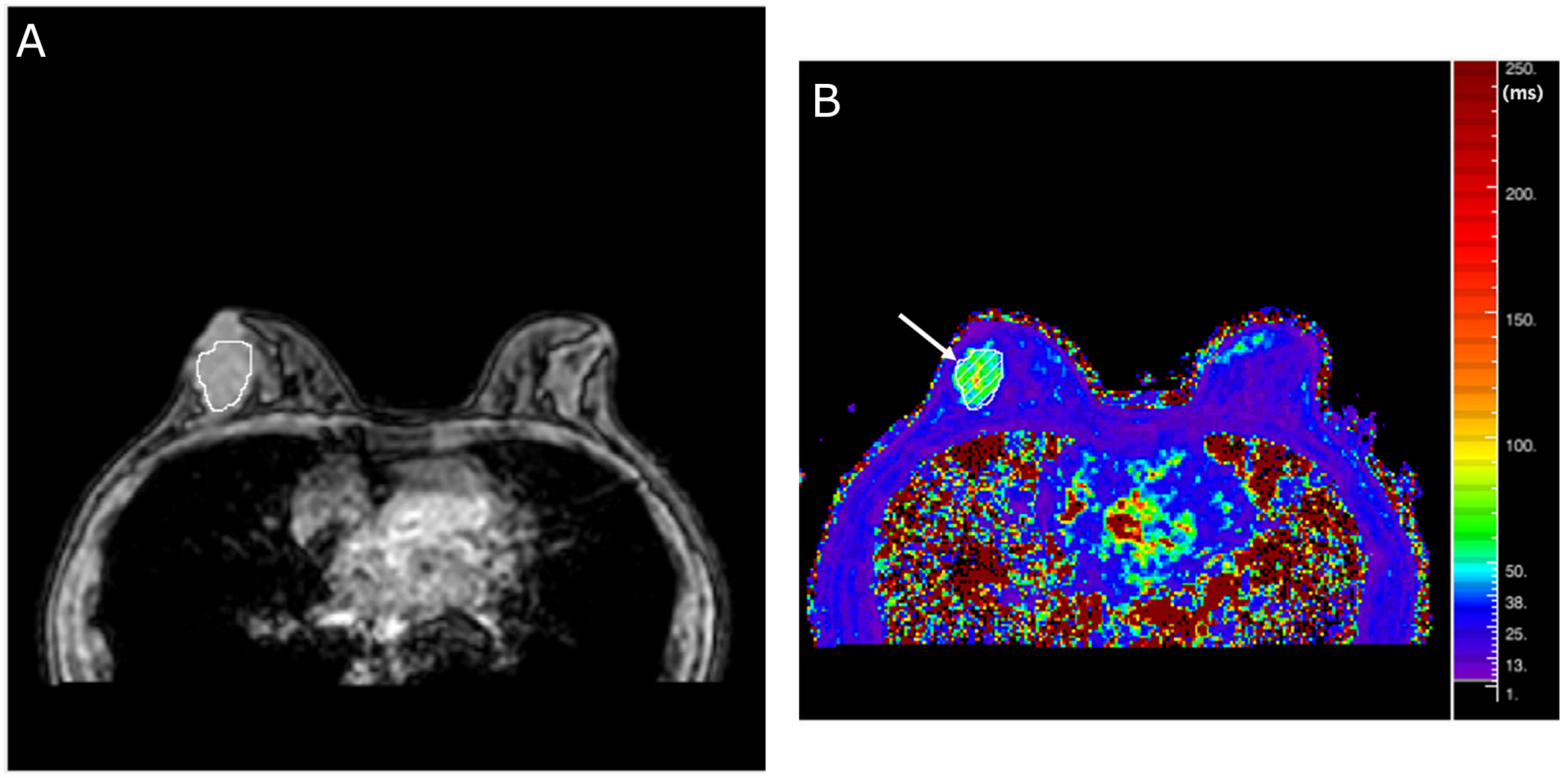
(A) Axial T2*-weighted image and (B) color-coded R2* map from BOLD-MRI of a 34-mm-diameter grade 1 IDC (T2N1M0) in a 48-year-old woman. The R2* map shows heterogeneous signal intensity in the tumor (arrow). The red color represents the highest R2* values, indicating the highest concentration of deoxyhemoglobin; the blue color represents the lowest R2* values, indicating the lowest concentration of deoxyhemoglobin. The mean R2* value was 56.08 Hz.
